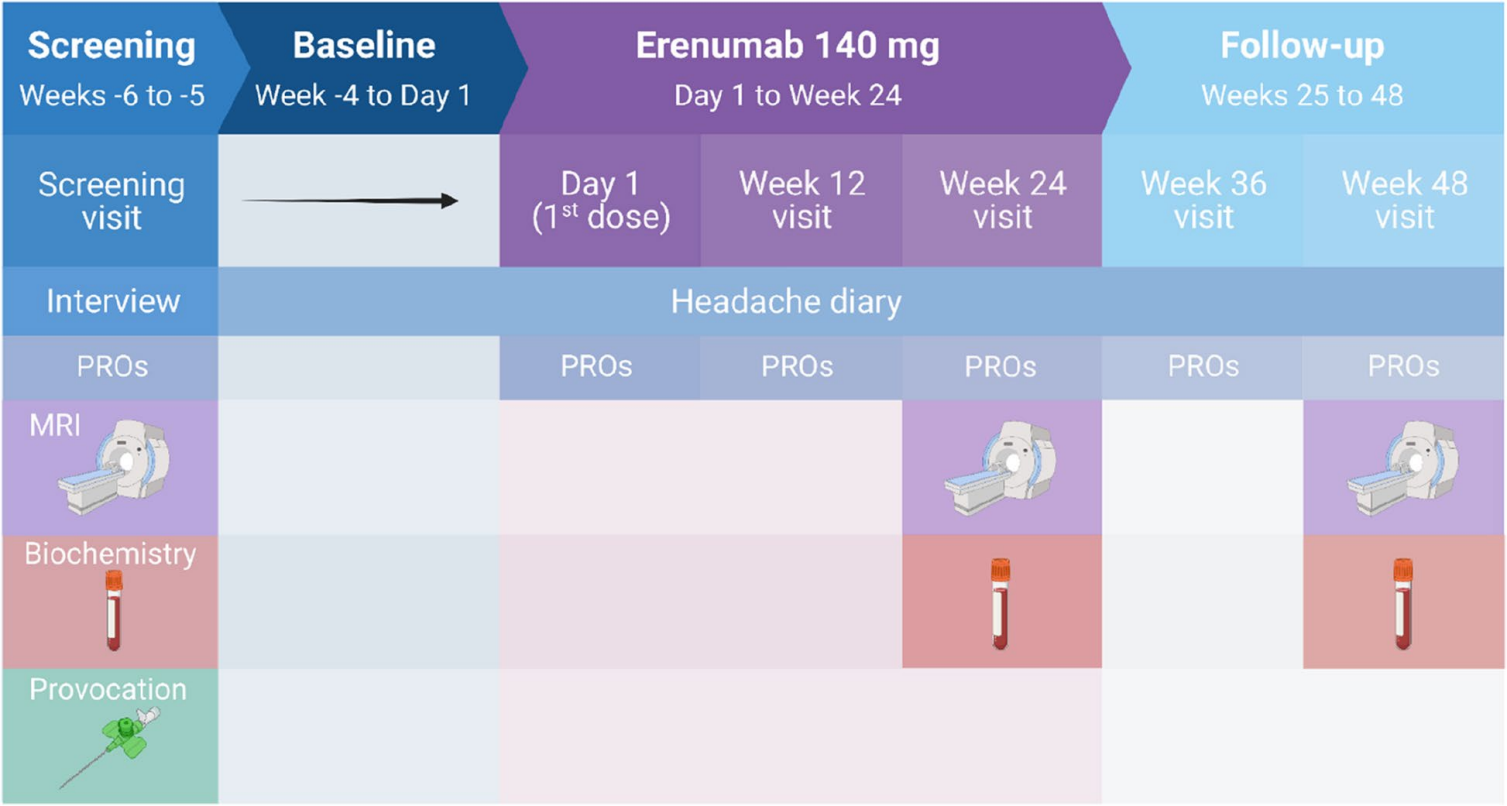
Study overview. All participants were enrolled in the REFORM Clinical Core, whilst participation in the Biochemistry, MRI, and Provocation Core was optional. The Clinical Core consisted of four periods: a 2-week screening period (Weeks -6 to -5), a 4-week baseline period (Week -4 to Day 1), a 24-week treatment period (Day 1 to Week 24), and a 24-week follow-up period without treatment (Weeks 25 to 48). Participants were evaluated for study eligibility and underwent a semi-structured interview at the Screening visit (Weeks -6 to -5). Those who participated in the Biochemistry and/or MRI Cores had their blood samples collected at three different timepoints: Screening (Weeks -6 to -5), Week 24 (± 2 weeks), and Week 48 (± 4 weeks). For participants who were also involved in the MRI or Provocation Core, blood samples were obtained on the day of their first MRI scan or the day of the CGRP-provocation experiment. Participants were asked to complete nine patient-reported outcomes at Screening, Day 1 (1^st^ dose), and every 12 weeks throughout the treatment period and the subsequent follow-up period without treatment. Healthy controls underwent blood sample collection and MRI scans at one scheduled site visit. Figure was created using BioRender.com
